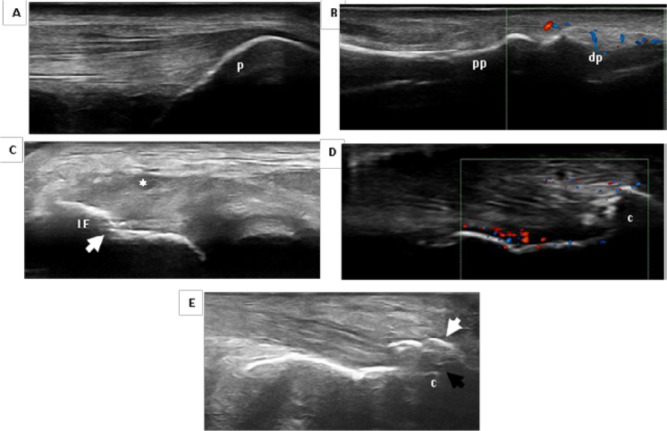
(A) Longitudinal ultrasonographic image of the quadriceps tendon shows a normal aspect of the enthesis. (B) Longitudinal ultrasonographic image of the deep flexor tendon of the 3rd finger shows power Doppler signal. (C) Longitudinal ultrasonographic image of the common extensor tendon insertion shows bone erosions (white arrow) abnormal thickening and hypoechogenicity (asterisk). (D) Longitudinal ultrasonographic image of the calcaneal tendon shows power Doppler signal. (E) Longitudinal ultrasonographic image of the calcaneal tendon shows enthesophyte (white arrow) and bone erosion (black arrow). p: patella ; md: medial phalange; dp: distal phalange; LE: lateral epicondyle; c: calcaneus.
